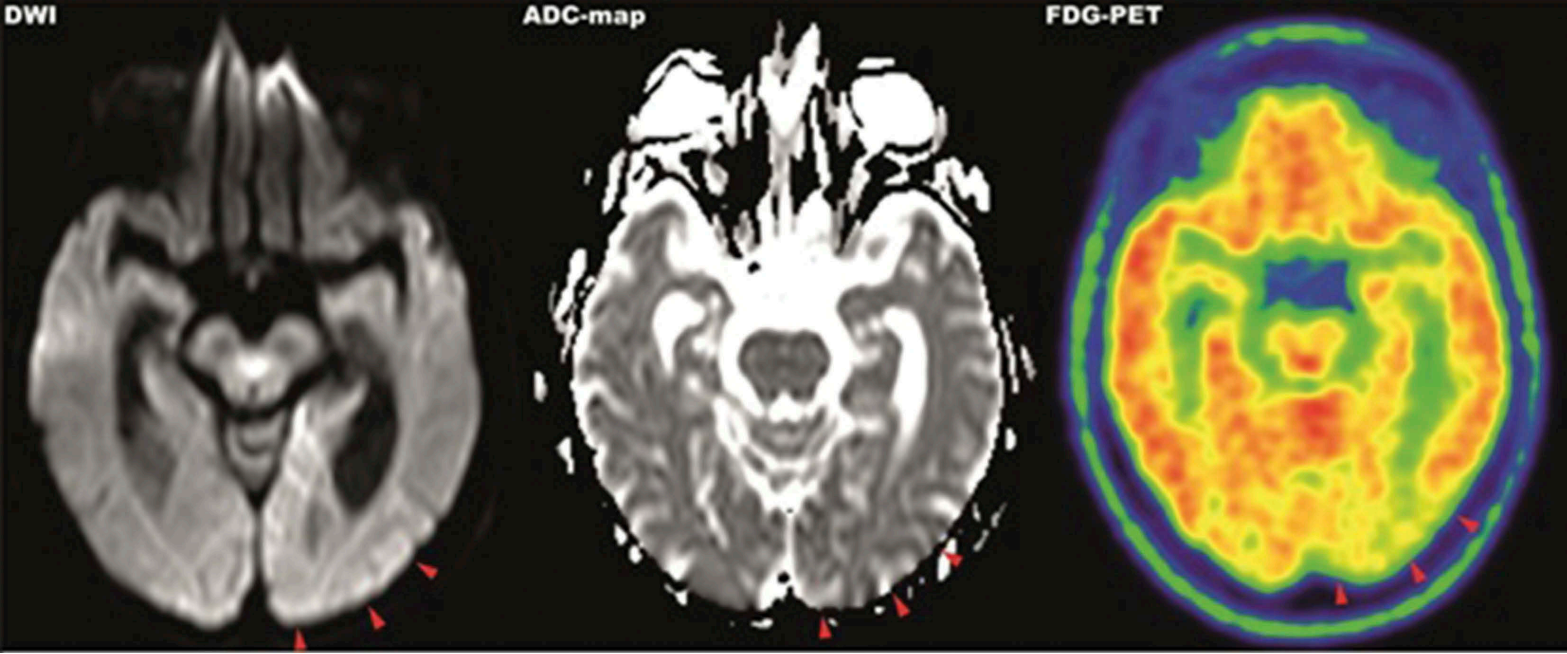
MRI-DWI (left), ADC-map (middle) and ^18^F-FDG-PET (right) imaging of parietooccipital cortex. MRI imaging showed hyperintense signals in the left occipitoparietal cortex in diffusion-weighted imaging with correlating hypointense signals in the ADC-map. The same area presents hypometabolic in 18^F^-FDG-PET imaging.
